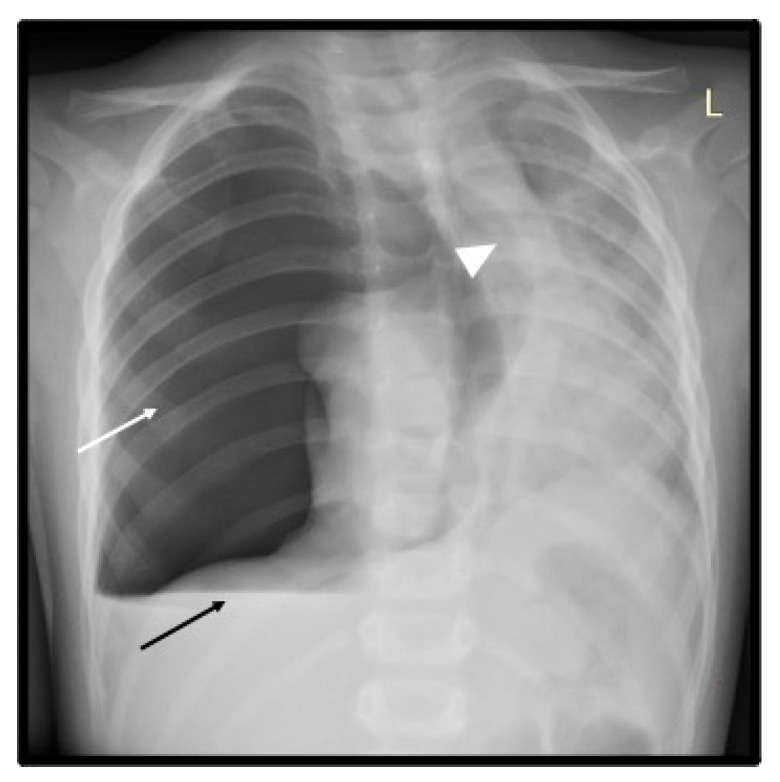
Day 7 of hospital admission. Frontal chest X-ray reveals a large right pneumothorax (white arrow) with a marked shift of the mediastinum to the left (white arrowhead) as demonstrated by the leftward deviation of the trachea and cardiac silhouette and small hydrothorax with air-fluid level (black arrow).
